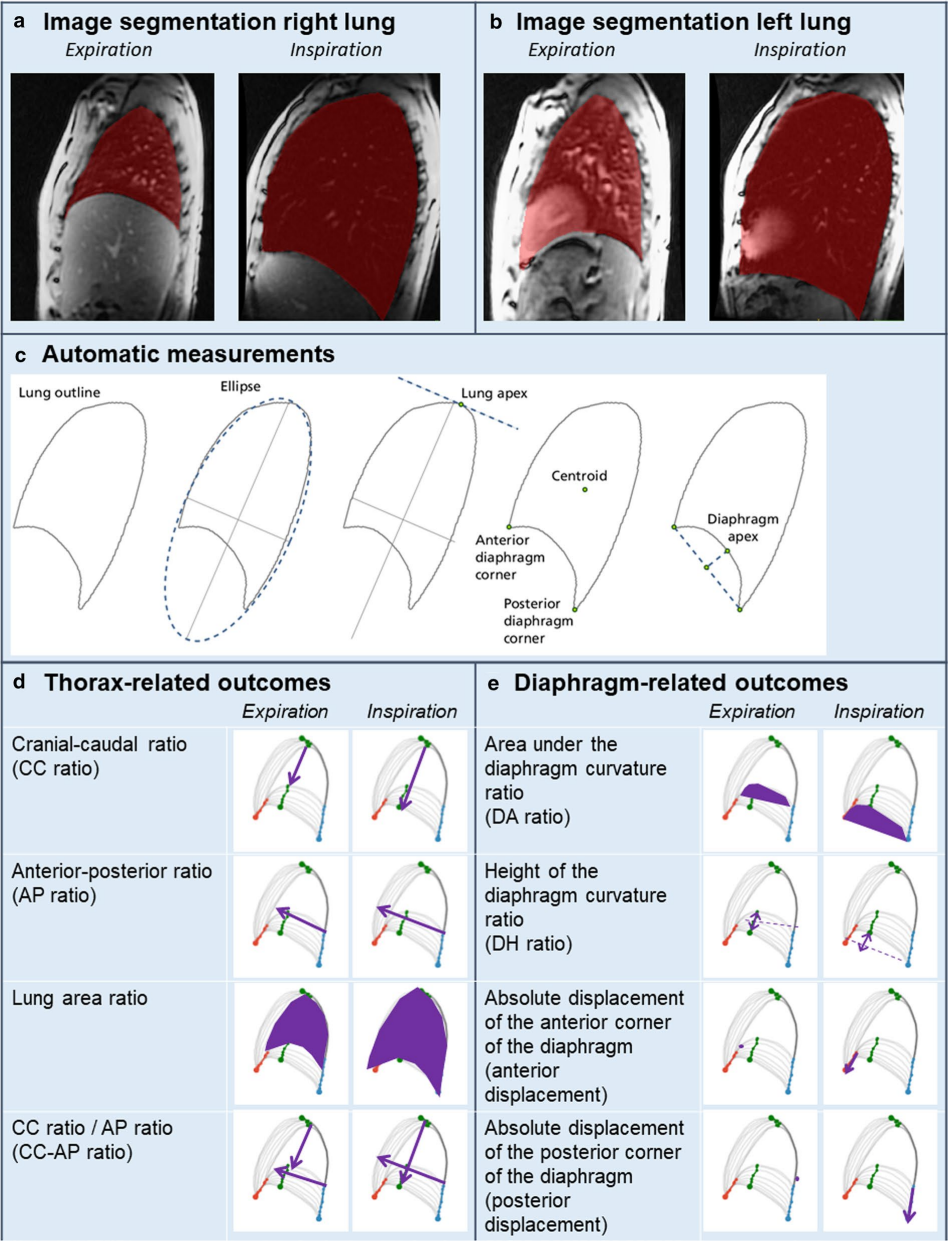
MRI measurements and outcomes. a, b Examples of manual image segmentation in the right and left lung at expiration and inspiration in an healthy control. c Steps of the automatic algorithm to define reference points to calculate the different outcome measures. d and e Thorax- and diaphragm-related outcomes. The grey lines depict the outlines of the lung in consecutive images from end expiration to end-inspiration. The red point indicates the anterior corner of the diaphragm and the blue point the posterior corner of the diaphragm. The green points indicate displacement of the lung apex and diaphragm apex. Purple arrows and purple areas indicate the outcome measurements
